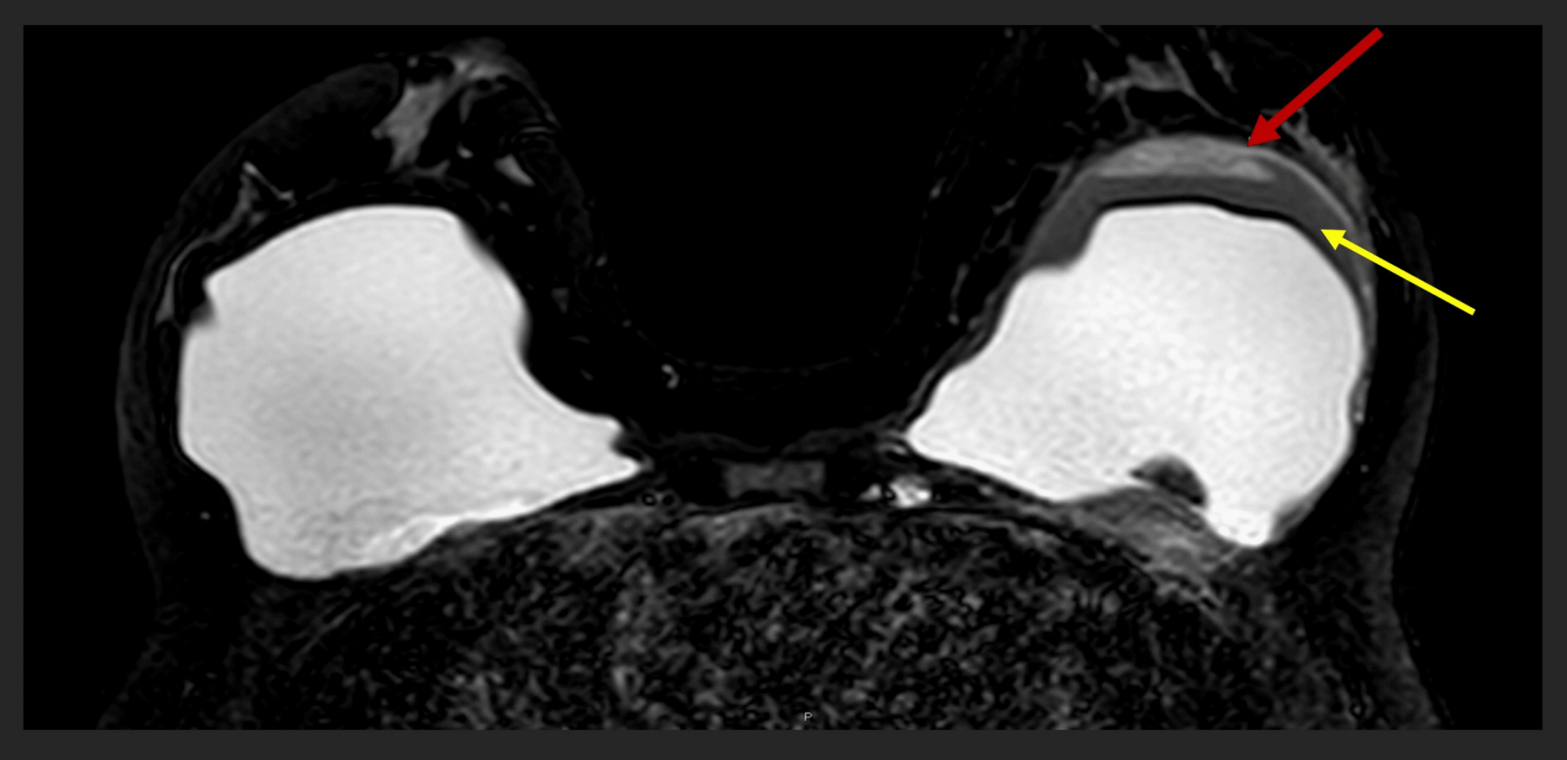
MRI breasts revealed intra-capsular rupture with silicone (red arrow) floating in the peri-implant effusion (yellow arrow) on the left breast. MRI: magnetic resonance imaging
